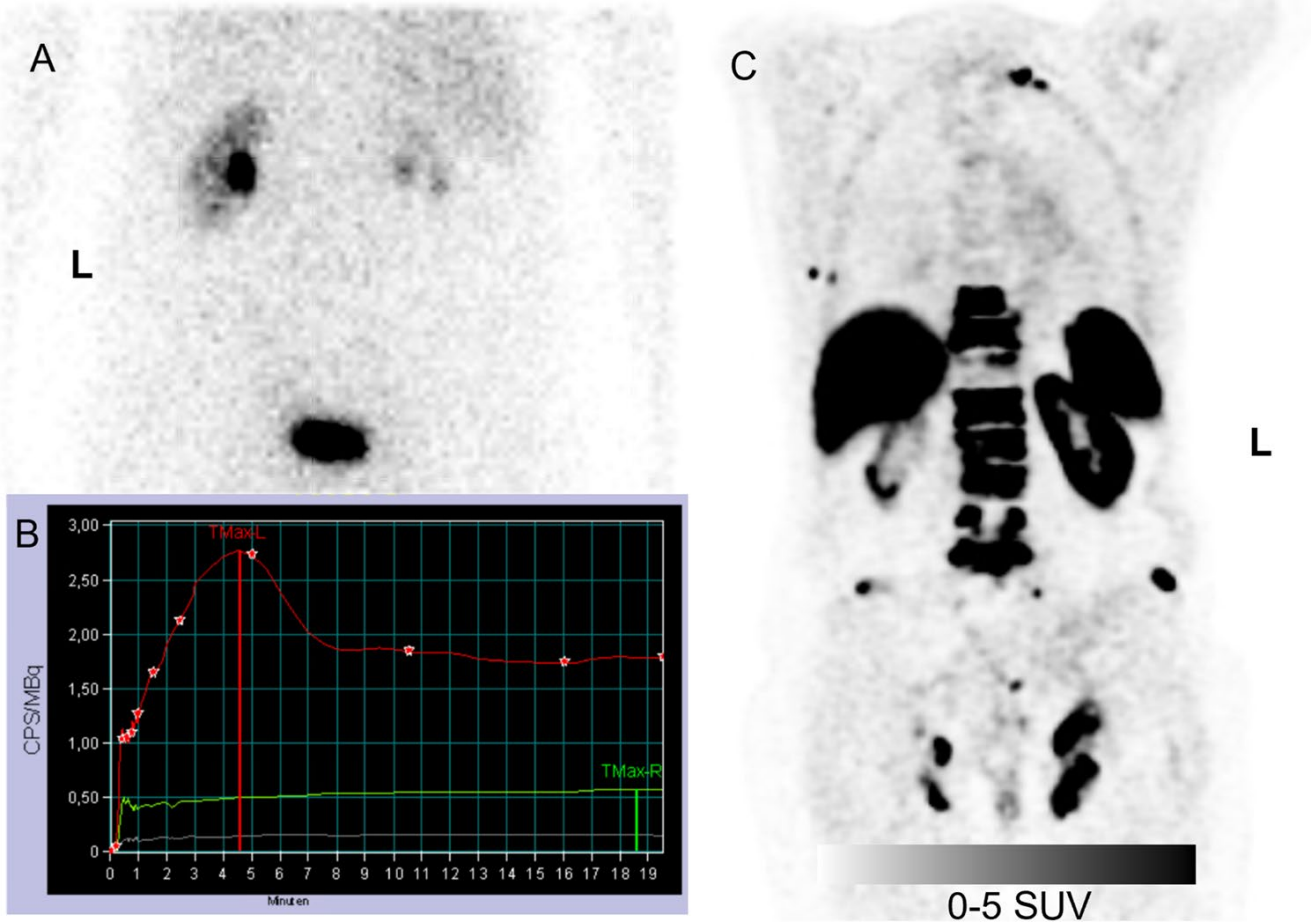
(A) [^99m^Tc]Tc-MAG3 scintigraphy acquired 20 min post-injection after administration of 112 MBq, with the corresponding renogram curves (B) of both kidneys shown below. (C) Coronal PET/CT images acquired 62 min post-injection after administration of 340 MBq [^18^F]F-flotufolastat are shown on the right. Split renal function calculated from both modalities shows concordant results, with MAG3 scintigraphy indicating a functional contribution of 94% for the left kidney and 6% for the right kidney, and PET-based analysis showing 91% and 9%, respectively ^18^F = Fluorine-18; MAG3 = mercapto-acetyltriglycine; PET = positron emission tomography; SRF = split renal function; ⁹⁹ᵐTc = Technetium-99 m
